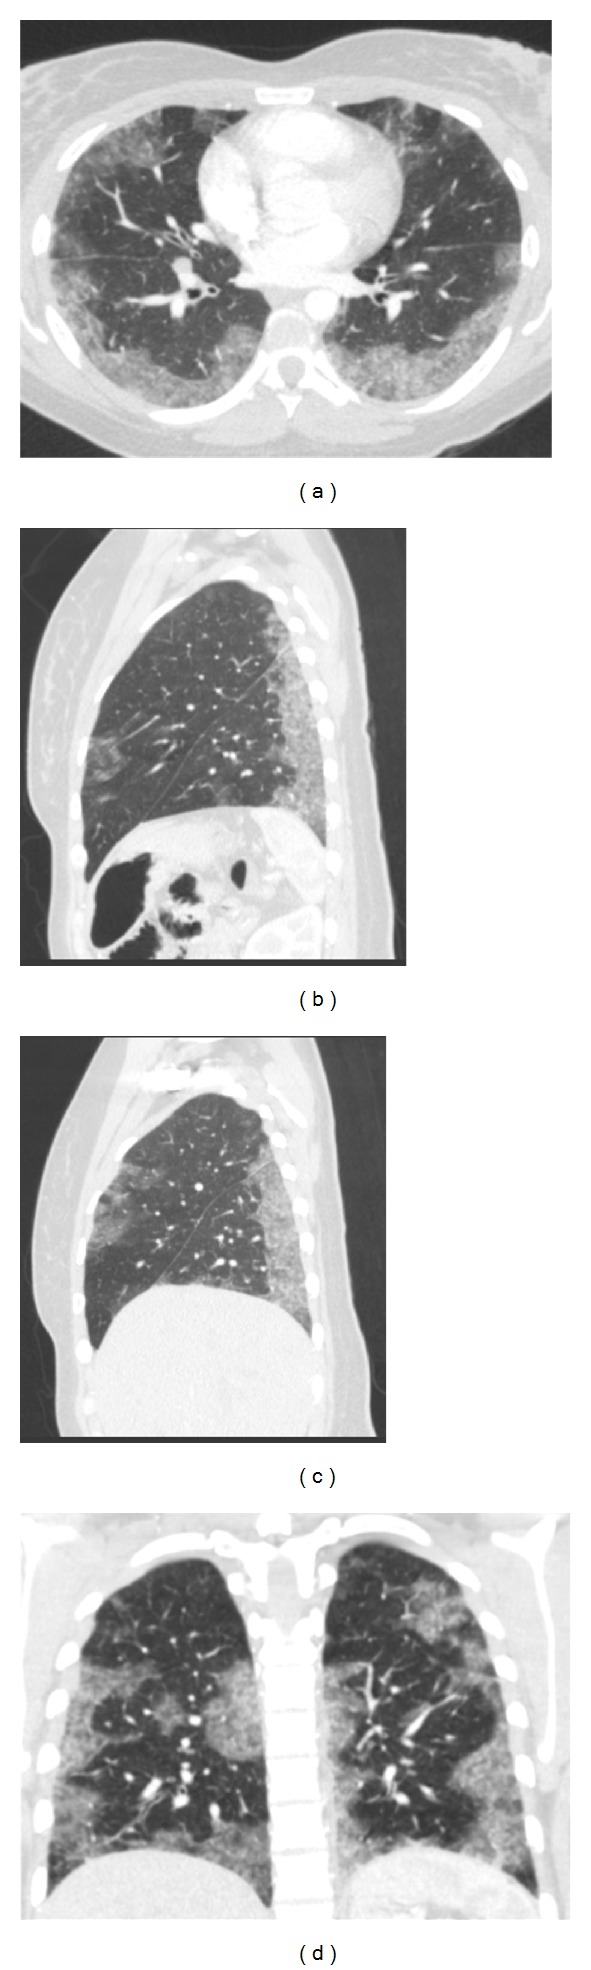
A 30-year-old female after illicit silicone injection. Findings: CT pulmonary angiography (axial (a), left sagittal (b), right sagittal (c) and coronal (d)) demonstrates diffuse, bilateral, ground-glass opacities greater on the right than on the left and predominantly at the lung bases.
